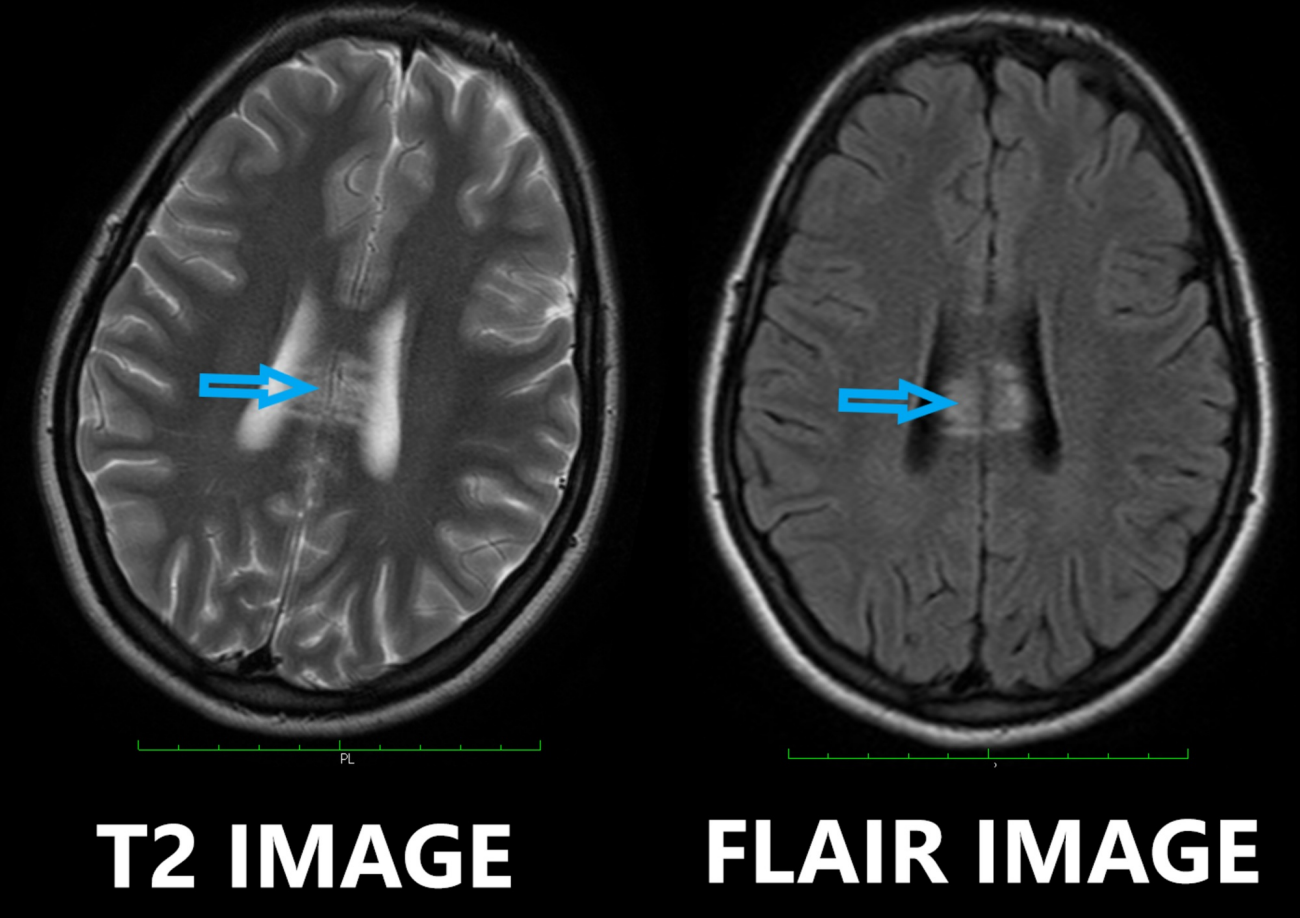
T2-weighted and fluid-attenuated inversion recovery (T2-FLAIR) axial MRI images showing multiple punctate lesions in the corpus callosum (blue arrows) MRI: magnetic resonance imaging
